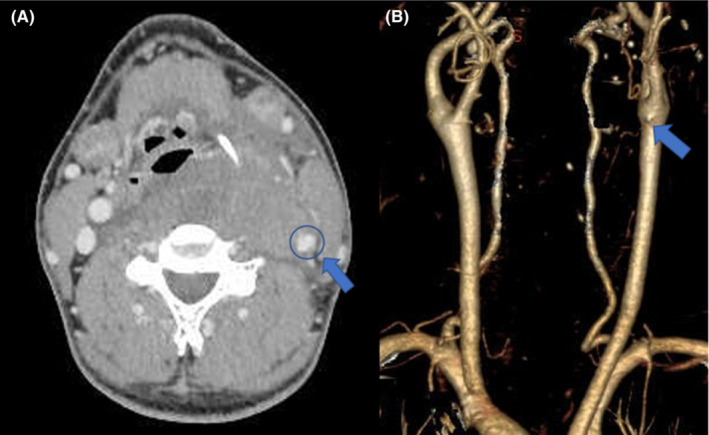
(A) A small extravasation that appeared to be a hemorrhage point was observed in one slice of the computed tomography image. (B) Postoperative three‐dimensional images also revealed that the same site was the source of the idiopathic hemorrhage
